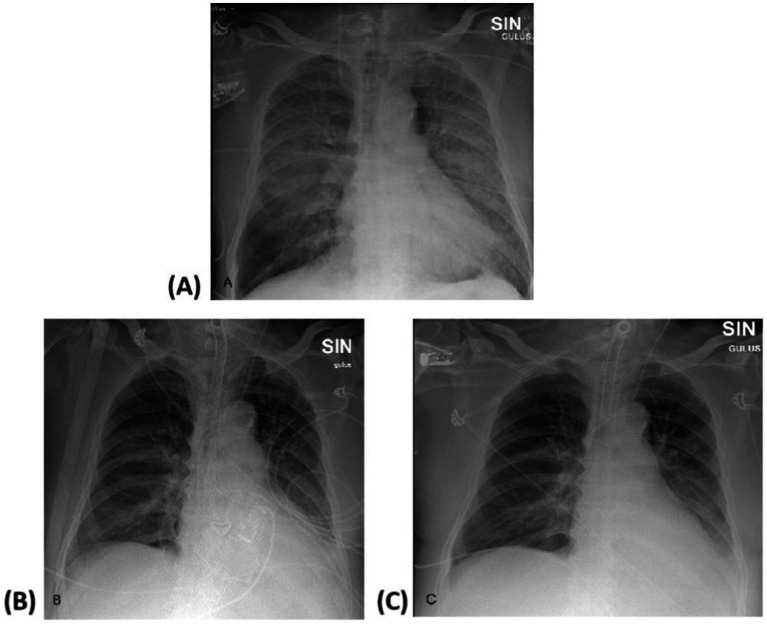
Portable chest X-rays. (A) X-ray taken on ICU day 2 (prior VV-ECMO cannulation). This radiograph demonstrates acute lung failure, with almost complete opacification of both lung fields. The bronchovascular pattern is accentuated, with uneven alveolar infiltrative changes bilaterally, more pronounced on the left side, suggesting pulmonary edema. The lung roots are homogenized, and the diaphragmatic domes are visible. There is no significant volume of contents in the pleural spaces. The heart and aorta shadows appear of normal size and configuration. (B) Day 8, before decannulation of VV-ECMO: The ECMO cannula is visible in the right internal jugular vein, with its tip reaching the distal third of the superior vena cava. Slight bronchovascular infiltrative changes are seen in the perihilar regions. Bilateral pulmonary ventilation has further improved, and the volume and intensity of atypical infiltrative changes have further decreased. (C) Day 9, after VV-ECMO decannulation: Compared to the previous examination, there is a significant improvement in bilateral lung ventilation, with a decrease in both the volume and intensity of atypical infiltrative changes.
